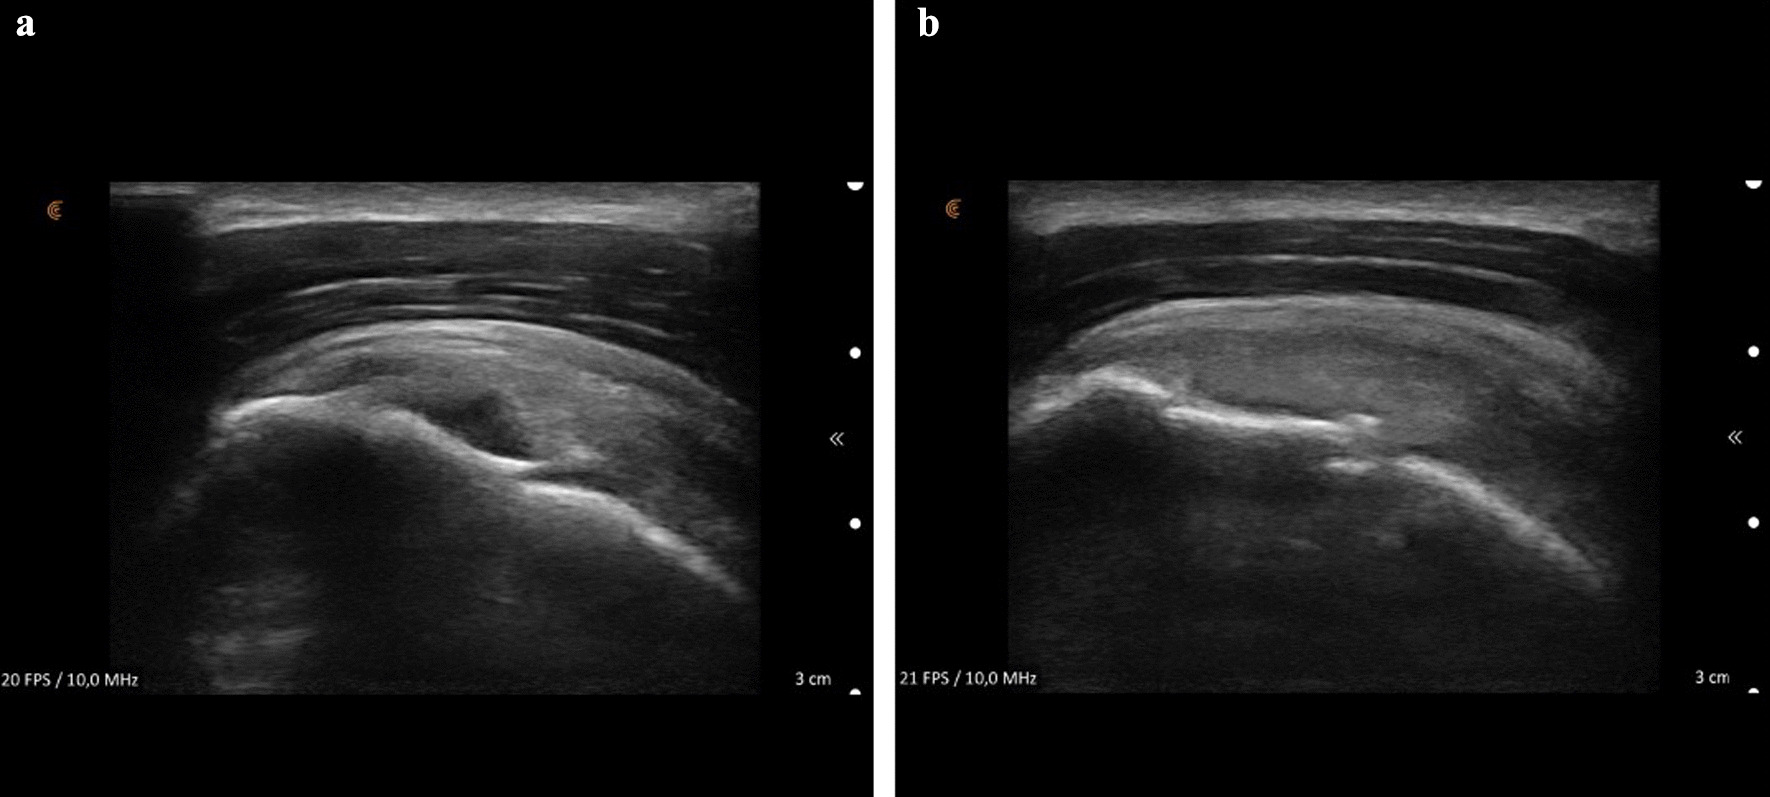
Ultrasound picture of the supraspinatus tendon of the left shoulder. First picture was taken before the treatment, showing a partial tear (a). The second picture was taken 18 days after the first treatment (b)
